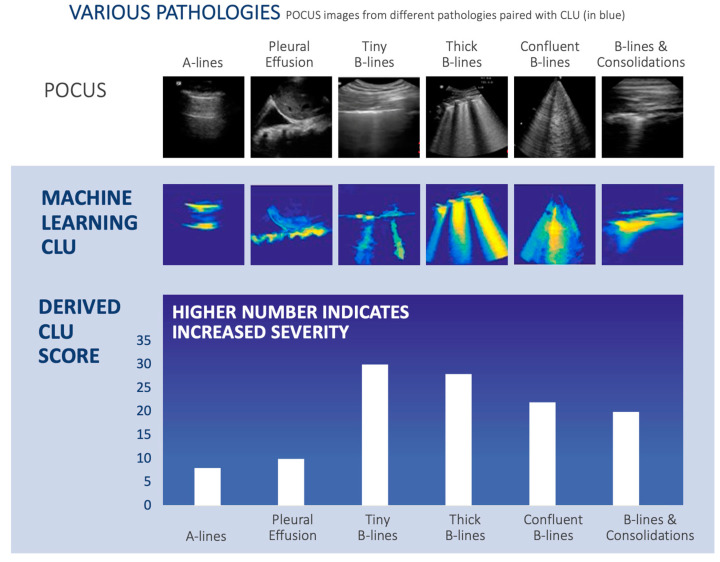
The ML-based POCUS CLU score. (Top row) Lung POCUS images in different pathologies in the “POCUS” row, with characteristic findings highlighted under the “machine leaning” row. Associated findings are color-coded, ranging from cyan to orange-red, with normal tissue and background in dark blue. (Bottom row) CLU score derived from POCUS. A normal-appearing CLU is ~10, whereas other findings increase the score above 10. POCUS = point of care ultrasound, CLU = calculated lung ultrasound.
